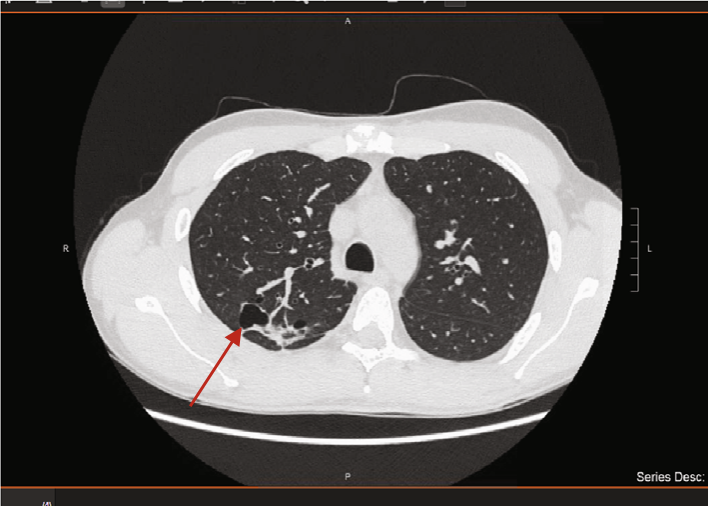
CT thorax at 6-month follow-up: residual small lung cavity at the right upper lobe.
